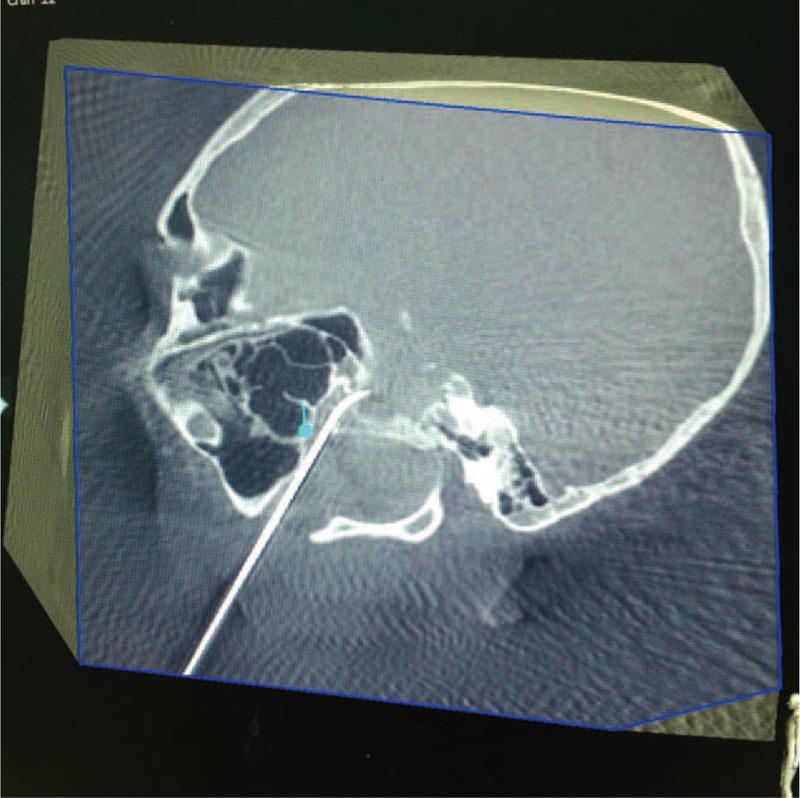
A lateral CT image showing the position of the needle in foramen rotundum. CT = computed tomography.
